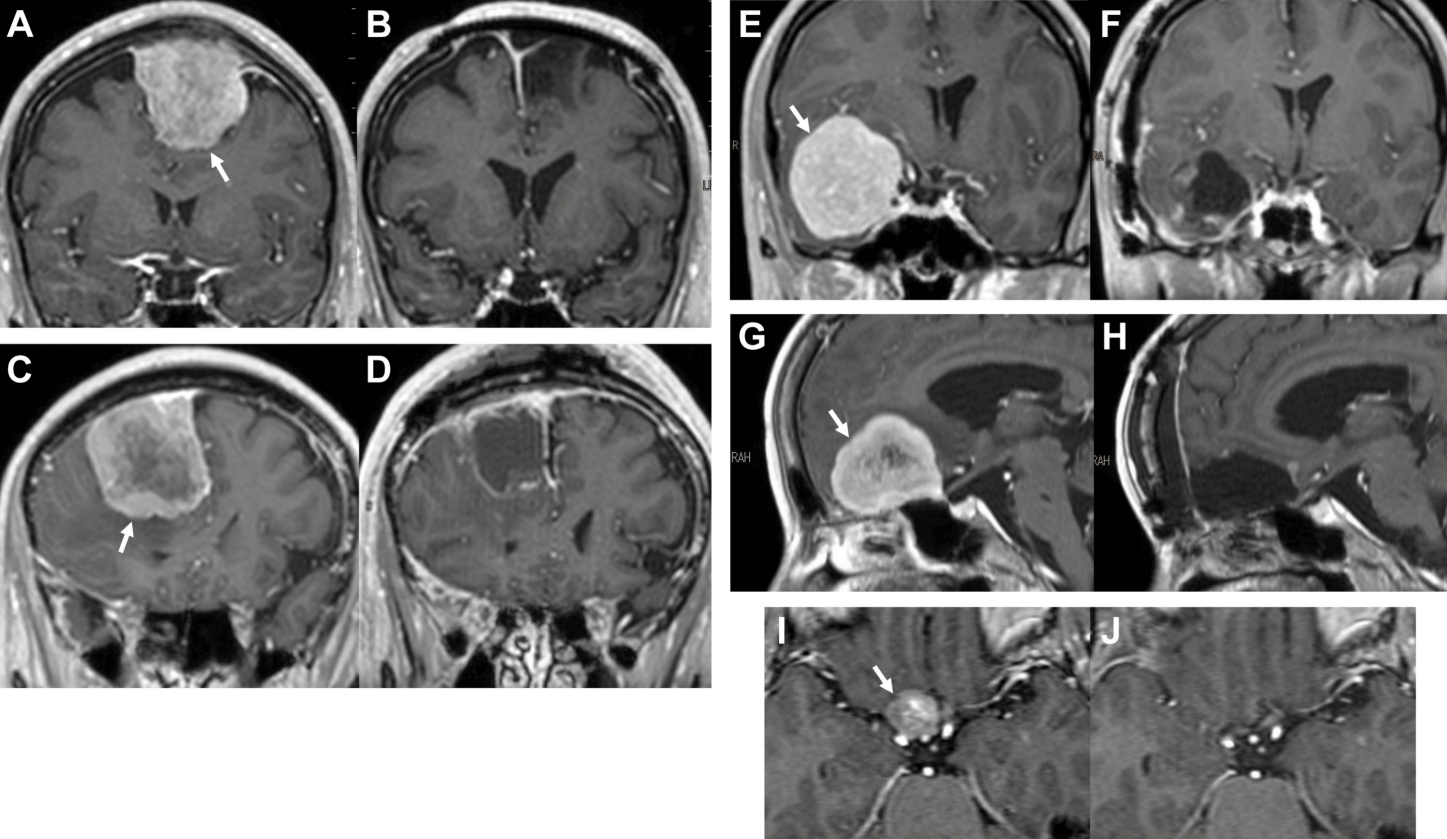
Representative magnetic resonance images (gadolinium-enhanced T1-weighted images). (A)-(B) Before and after surgery of case 2 in the presence of a left convexity-parasagittal meningioma (arrow). (C)-(D) Before and after surgery of case 3 in the presence of a right parasagittal-falx meningioma (arrow). (E)-(F) Before and after surgery of case 5 in the presence of a right middle fossa meningioma (arrow). (G)-(H) Before and after surgery of case 6 in the presence of an anterior fossa meningioma (arrow). (I)-(J) Before and after surgery of case 7 in the presence of a right tuberculum sellae meningioma (arrow).
